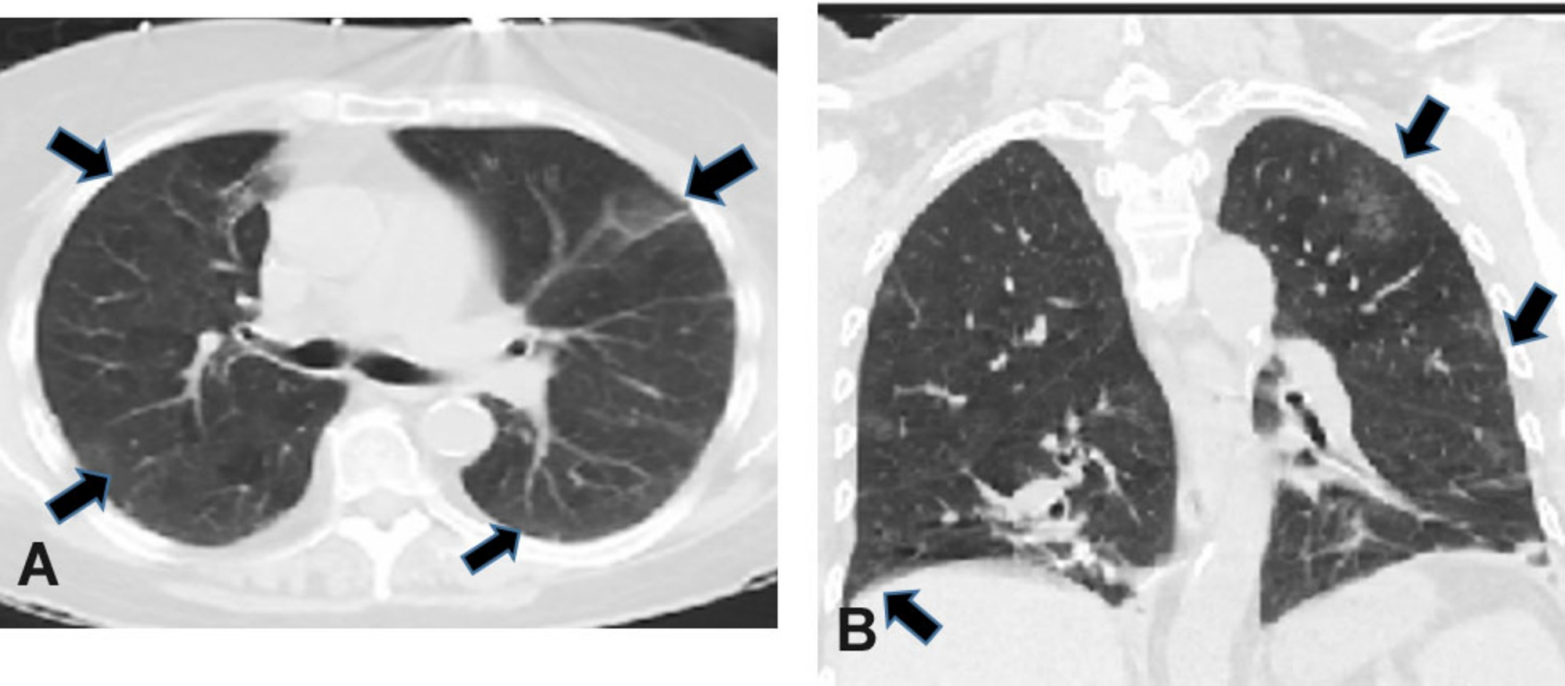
Computer tomography scan images of lungs. A, Axial CT with bilateral ground glass opacities (arrows) in the right and left upper lung lobes. B, Coronal CT with bilateral ground glass opacities (arrows) in the right and left upper and lower lung lobes.
